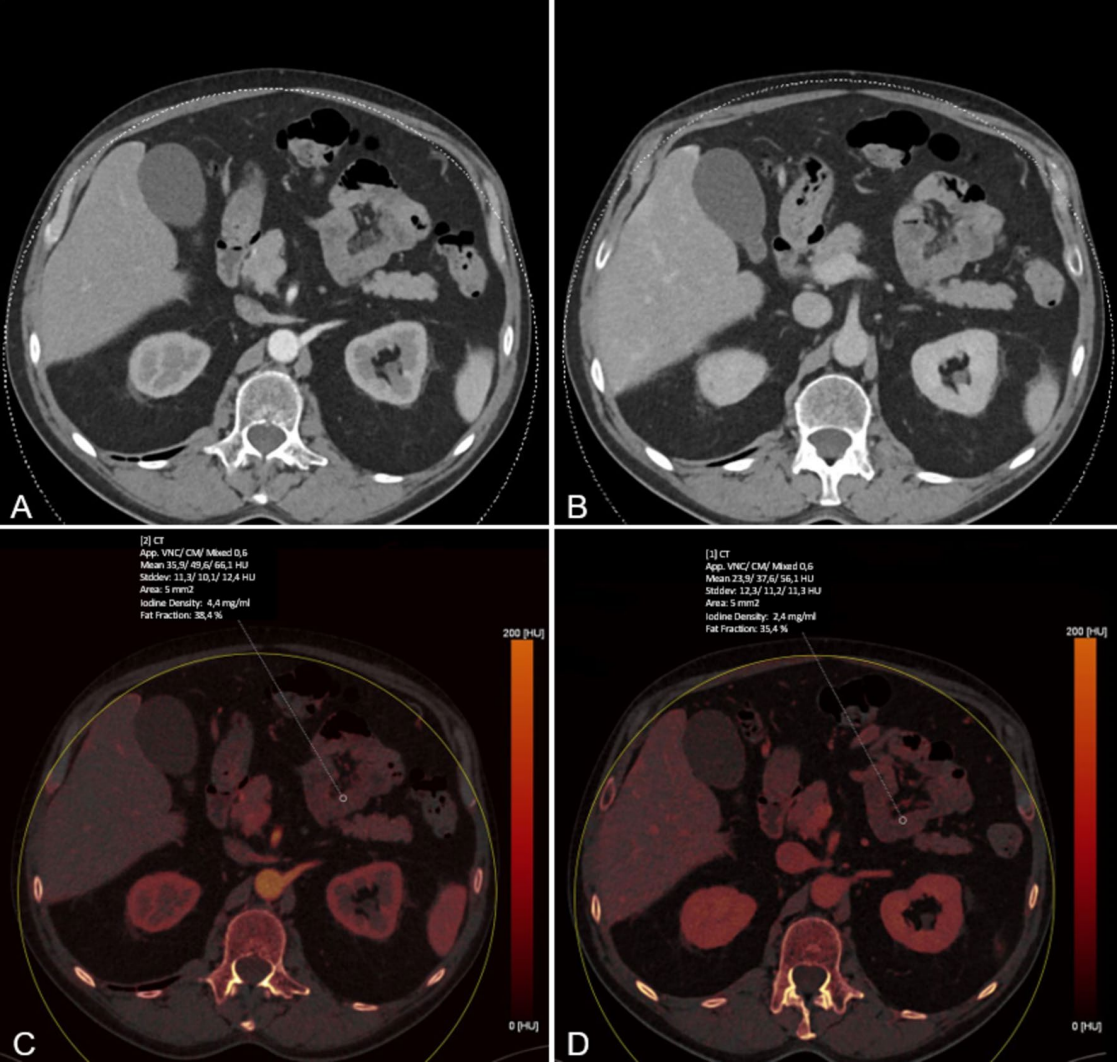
Conventional linearly blended late arterial (LA) and portal venous (PV) phase dual-energy CT scans (A and B, respectively) LA and PV iodine maps (C and D, respectively) were created using dedicated postprocessing software. Iodine concentration measurements in both phases and Hounsfield unit (HU) measurements were obtained by placing ten regions of interest (ROI, 5 mm^2^) in surgically proven ischemic and ten ROI in surgically proven non-ischemic bowel segments, respectively. Values were automatically computed by the postprocessing software.
